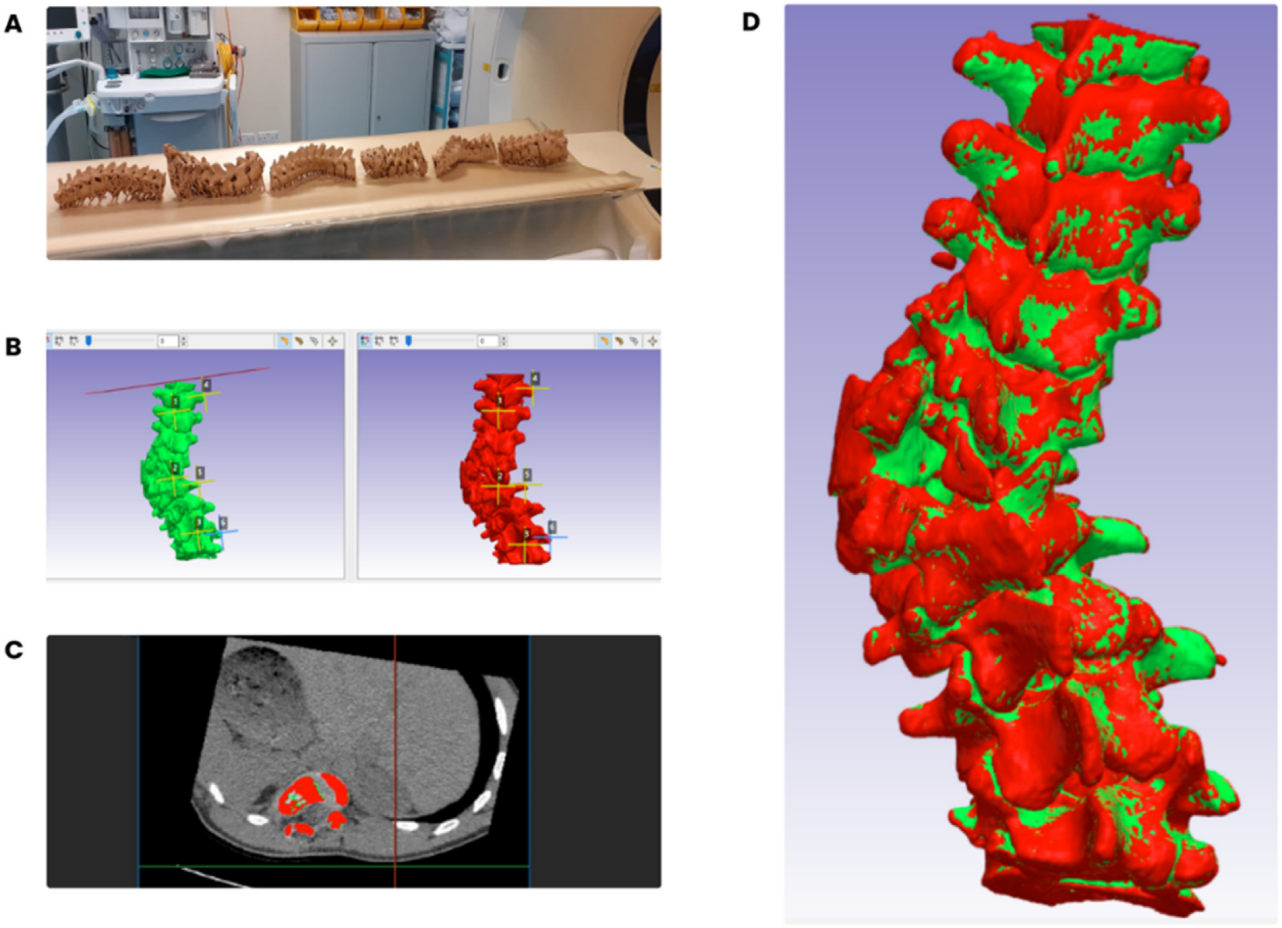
Superimposition and surface deviation analysis using Simpleware ScanIP: (A) CT-scan of 3D printed spinal models with region of deformity of interest, (B) six corresponding landmark placements on the patient ‘ground-truth’ (red) and model (green) virtual 3D reconstructions (C) axial view of superimposed images (D) final superimposition of 3D reconstructions
